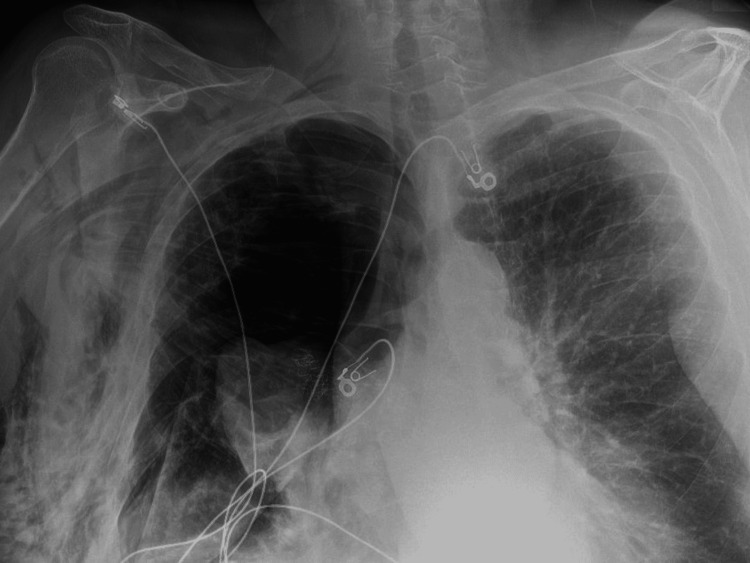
Chest radiograph showing right-sided pneumothorax following removal of malpositioned chest tube
